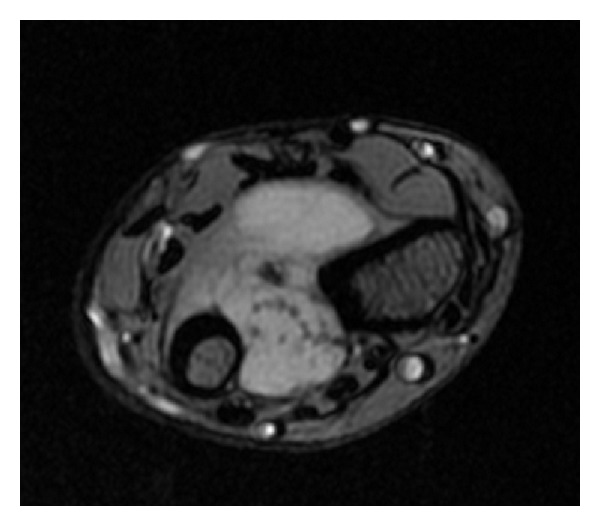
MRI of the left distal radioulnar joint showing calcified nodules consistent with synovial osteochondromatosis.
